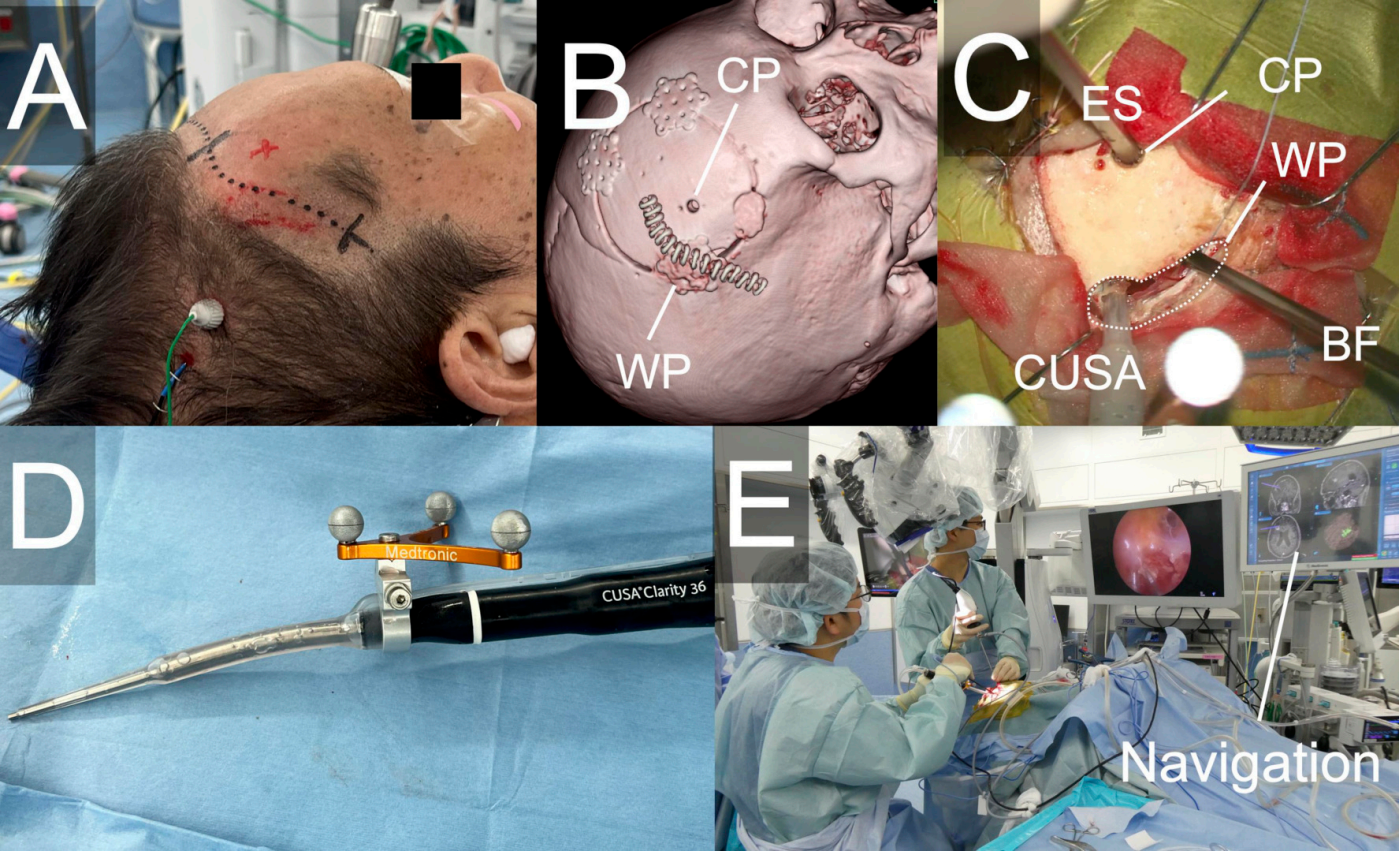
Operative setups and used instruments. (A) Skin incision utilizing part of the previous surgical incision. (B) Postoperative CT image showing the skin incision and the camera port. (C) Setup of the camera and surgical instruments during manipulation. (D) Optical navigation integrated with the CUSA. (E) Real-time navigation enabling simultaneous tumor resection and positional confirmation. CT, computed tomography; CP, camera port; WP, working port; ES, endoscope; BF, bipolar forceps.
